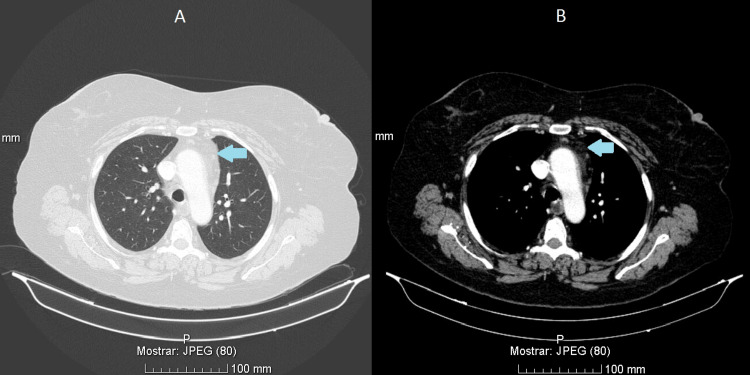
Chest computed tomography showing a marked reduction in the size of mediastinal and hilar lymph nodes, currently measuring less than 1 cm, with no criteria for lymphadenopathy (A: lung window; B: mediastinal window)
